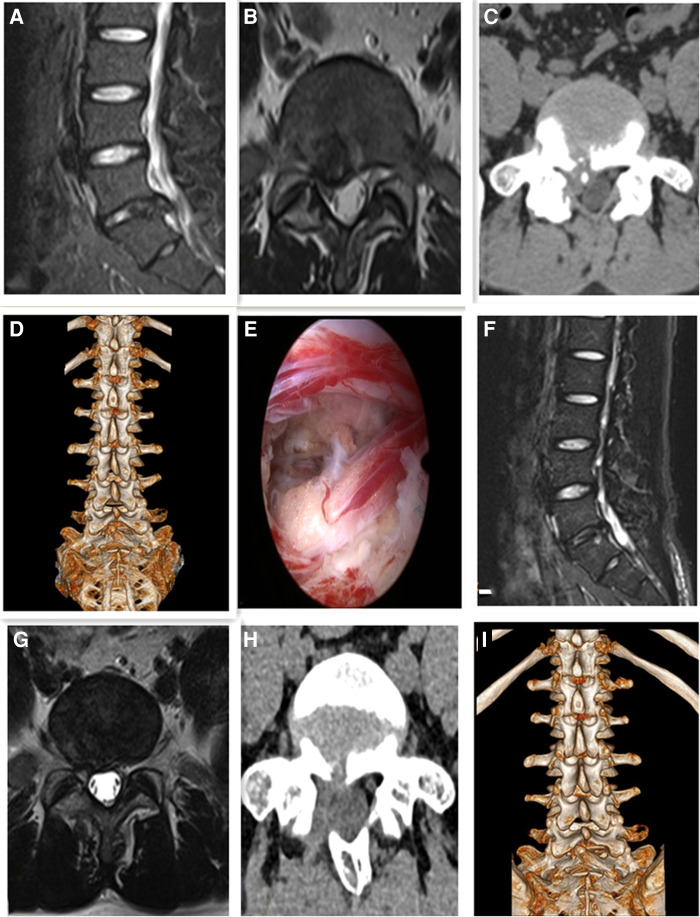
Male, 25 years old, L5/S1 lumbar disc herniation with posterior apophyseal ring separation. (A) Preoperative sagittal MR image showed L5/S1 lumbar disc herniation; (B) Preoperative axial MR image showed herniated lumbar disc compressed nerve root and dural sac; (C) Preoperative axial CT image showed separated bony fragment of vertebral body; (D) Preoperative 3D-CT image; (E) Intraoperative image after complete neural decompression; (F) Postoperative sagittal MR image revealed the complete decompression of the spinal canal; (G) Postoperative axial MR image showed the complete removal of herniated disc and bony fragment; (H) Postoperative axial CT image showed the removal of the posterior bony fragment; (I) Postoperative 3D-CT image showed the lamina window and preservation of the facet joints.
